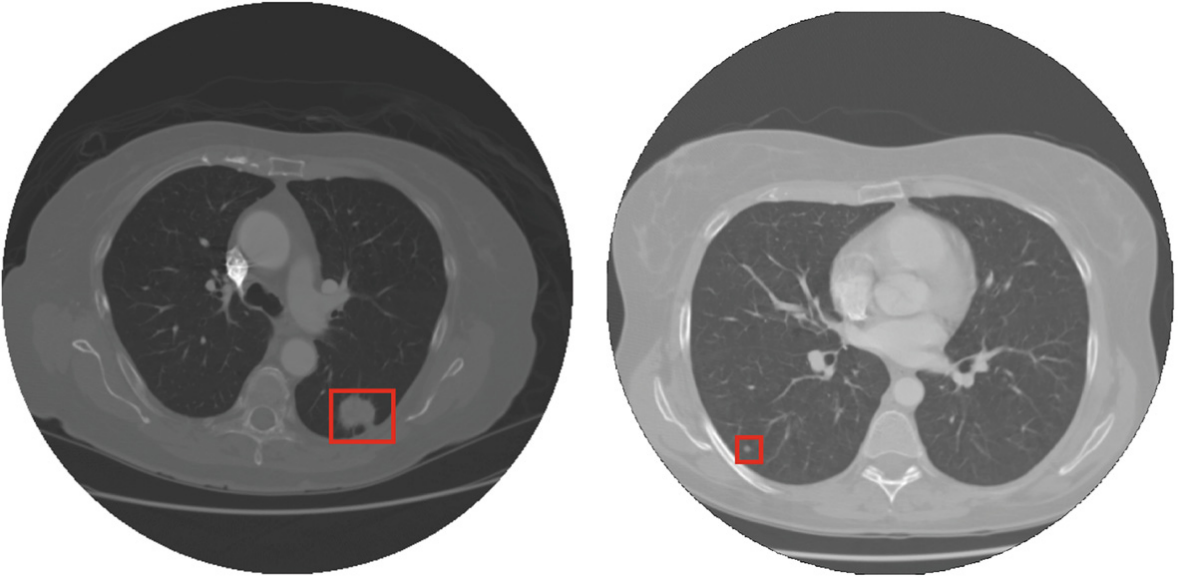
Transverse thoracic CT images of a patient with pulmonary nodules highlighted by square: juxtapleural nodule (left) and internal nodule (right). Computed tomography images of patients with pulmonary nodules obtained in the LIDC/IDRI Database.
